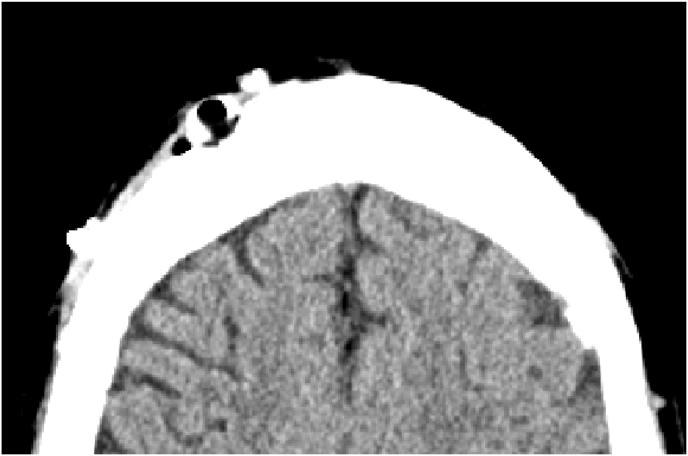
CT scan revealed the persistence of the air bubble.
